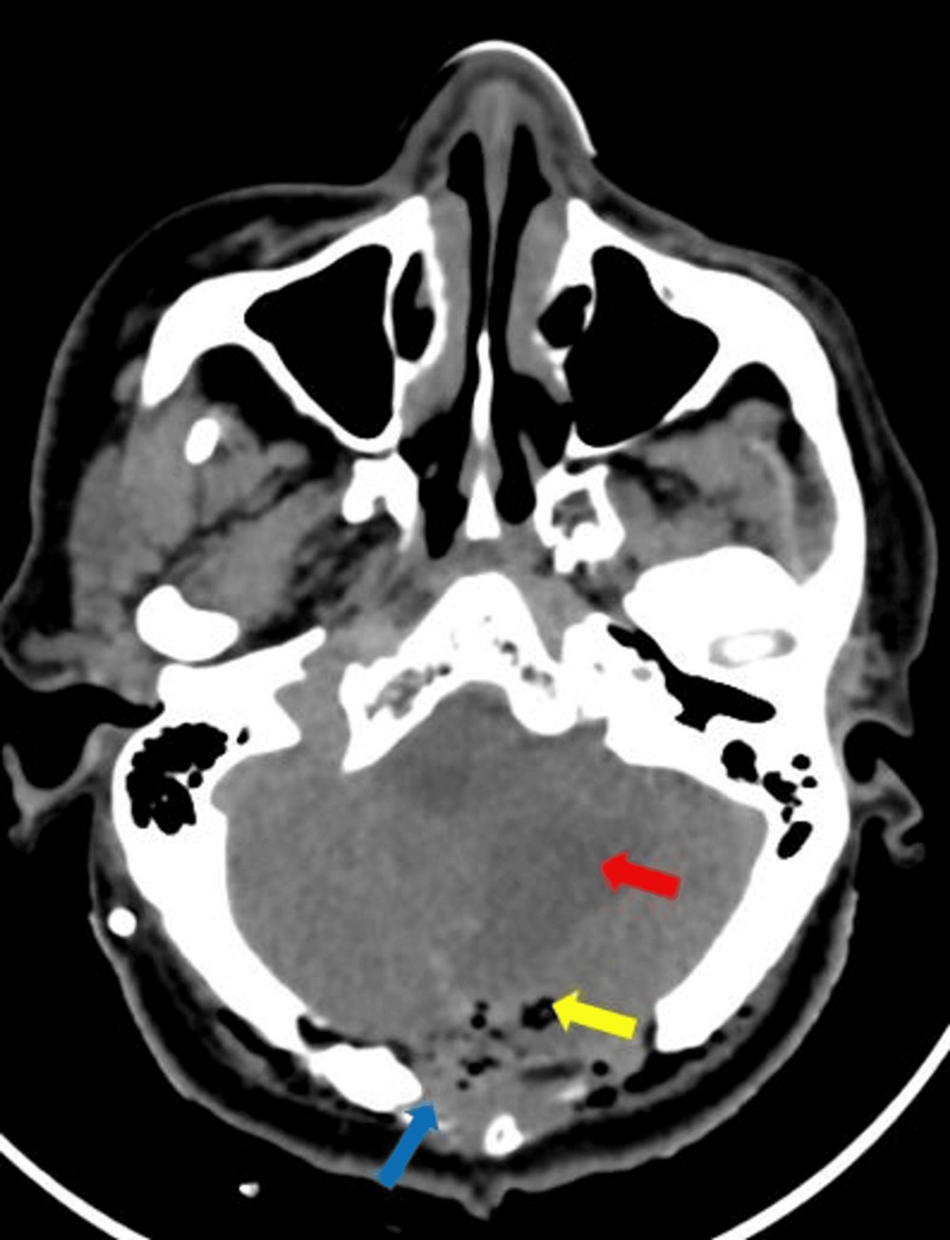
Post-operative non-contrast computed tomography This figure shows a craniotomy defect (blue arrow) in the occipital region with multiple air foci and hemorrhagic foci (yellow arrow) underneath the site of the defect, mostly postoperative changes. Hypodensity and edema are also seen in the right cerebellar hemisphere (red arrow)
